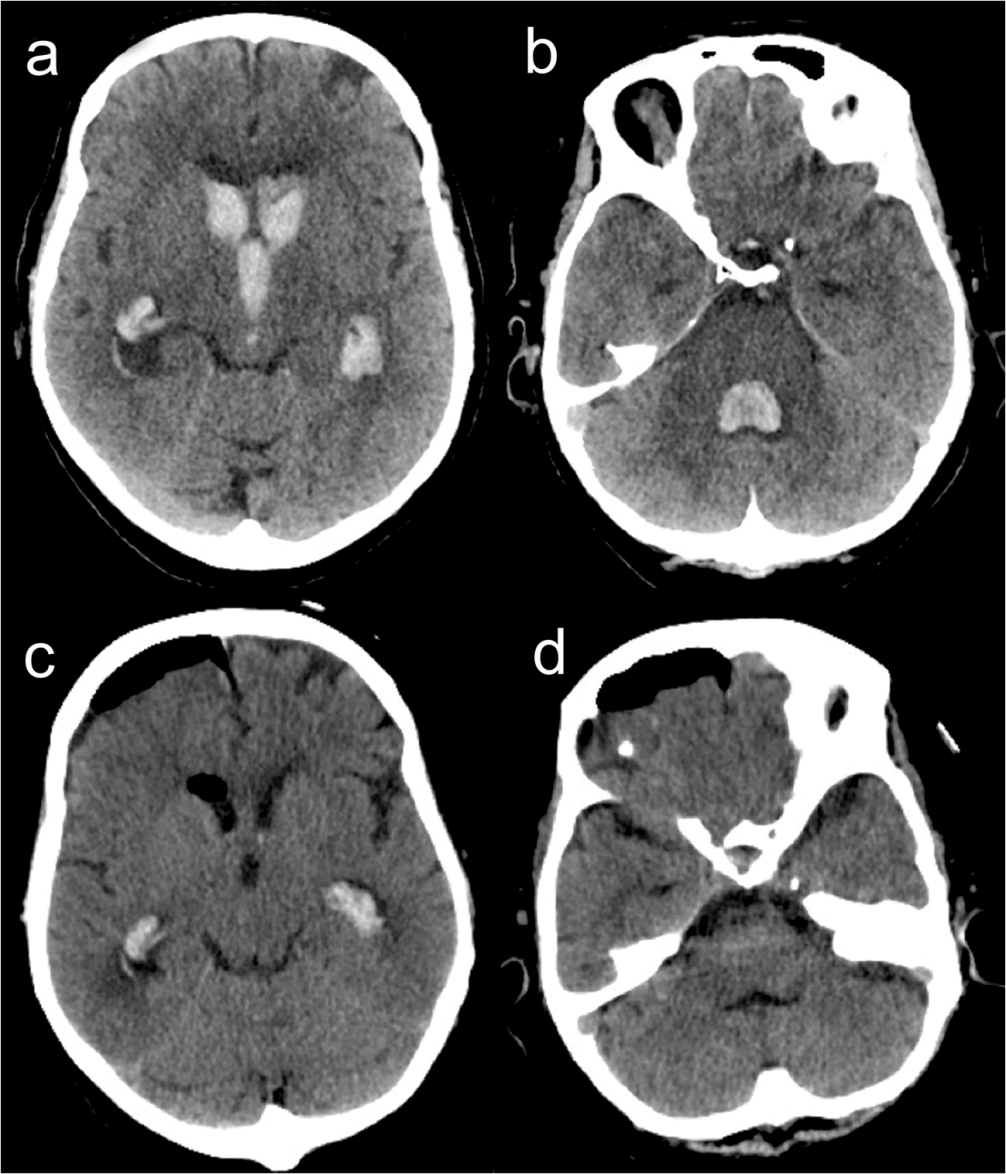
a, b Preop CT scan showing a tetraventricular hemorrhage in a patient affected by Moyamoya disease. c, d Immediate postop CT scan after endoscopic IVH aspiration
